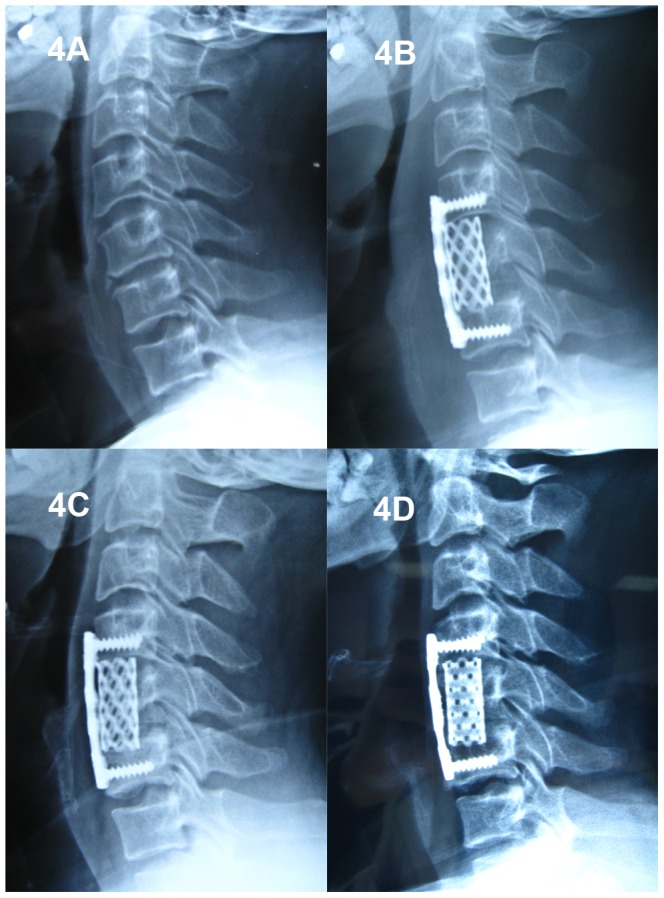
A 53-year-old male who underwent 1-level corpectomy with a titanium mesh cage used for cervical reconstruction. The preoperative cervical X-ray film (4A) and immediately postoperative lateral X-ray (4B) show C5 corpectomy and the titanium mesh cage used for reconstruction. The lateral X-ray one year postoperative (4C) and at the final follow-up (two years and six months; 4D) shows bony fusion between the graft and the adjacent endplates; nevertheless, marked cage subsidence was observed.
